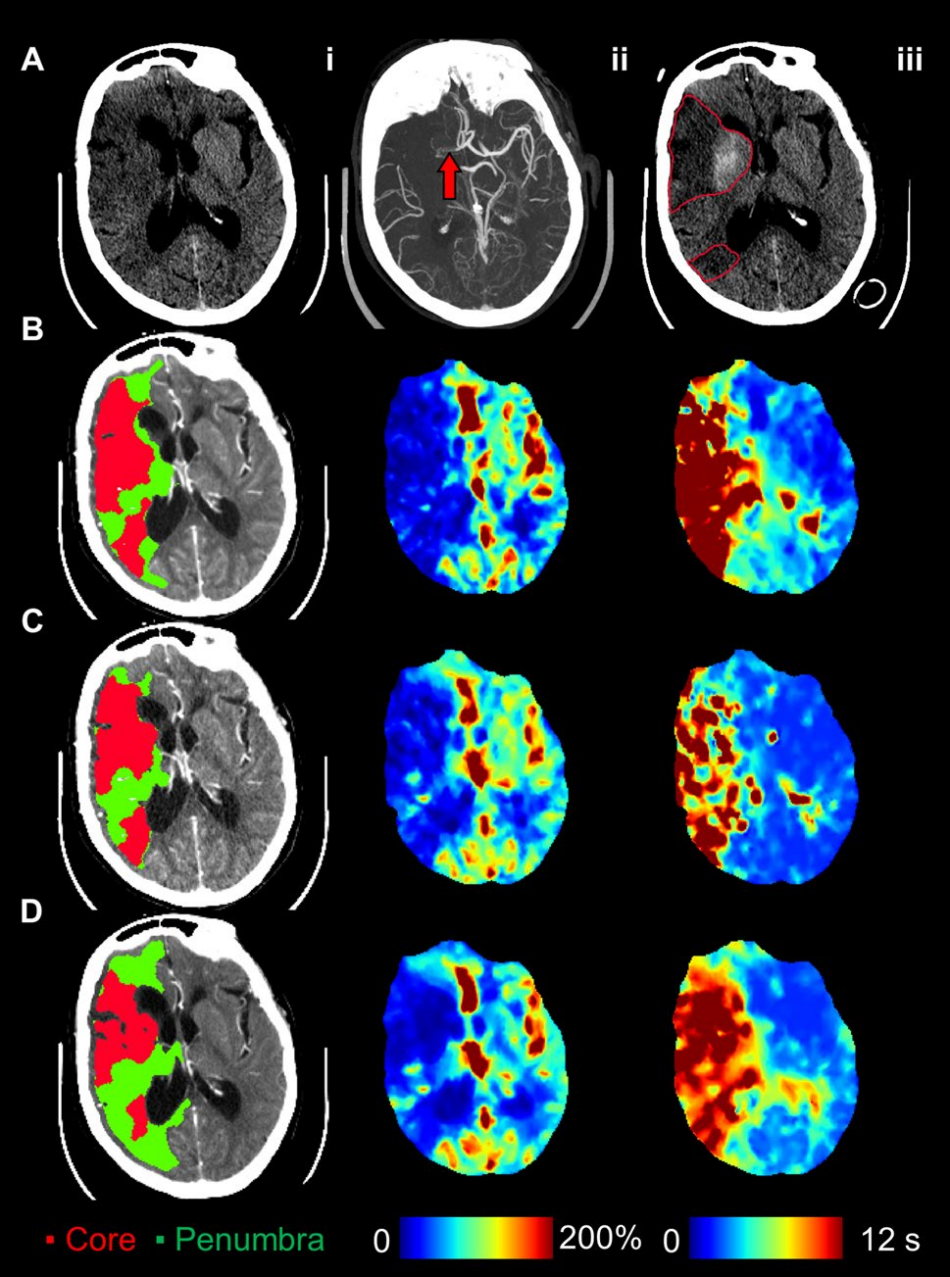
Patient presenting 5 h after stroke symptom onset with an occlusion of the right internal carotid to M1 middle cerebral artery and an NIHSS of 22. This patient did not receive reperfusion treatment. (A.i) Admission non-contrast CT shows early ischemic changes in the right MCA territory; (A.ii) maximum intensity projections of CT angiography with the red arrow indicating the occlusion site; (A.iii) follow-up non-contrast CT showing infarction and hemorrhage outlined in red. (B.i) CT perfusion ischemic core (red, 84.0 ml) and penumbra (green, 236.8 ml; mismatch ratio of 2.8) derived from cerebral blood flow (B.ii) and Tmax maps (B.iii). DEFUSE-3 mismatch was unfavourable by CT perfusion due to the large core volume. (C) Similarly, down-sampled CT perfusion (dCTP) indicated an unfavourable mismatch profile with an ischemic core and penumbral volumes of 71.7 ml and 205.3 ml, respectively (mismatch ratio 2.9). (D) Multiphase CT angiography-perfusion (mCTA-P) showed a similar ischemic lesion, but underestimated ischemic core and penumbra at 58.0 ml and 202.5 ml, respectively (mismatch ratio 3.5), leading to a favourable DEFUSE-3 mismatch profile and disagreement with CTP.
